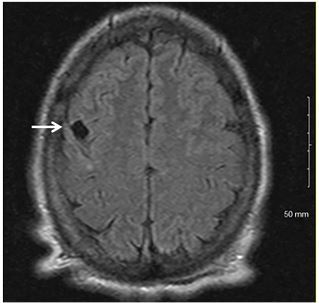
Magnetic resonance image of the brain revealing 12-millimeter right frontal lobe cerebrospinal fluid density (arrow).
